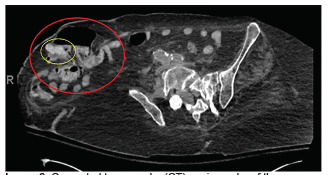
Computed tomography (CT) angiography of the abdomen and pelvis. This scan was performed hours after the CT (Image 2). It was found that inside the parastomal hernia (large red circle), contrast had appeared in this hernia, which was not previously visible on prior CT (circled yellow).
